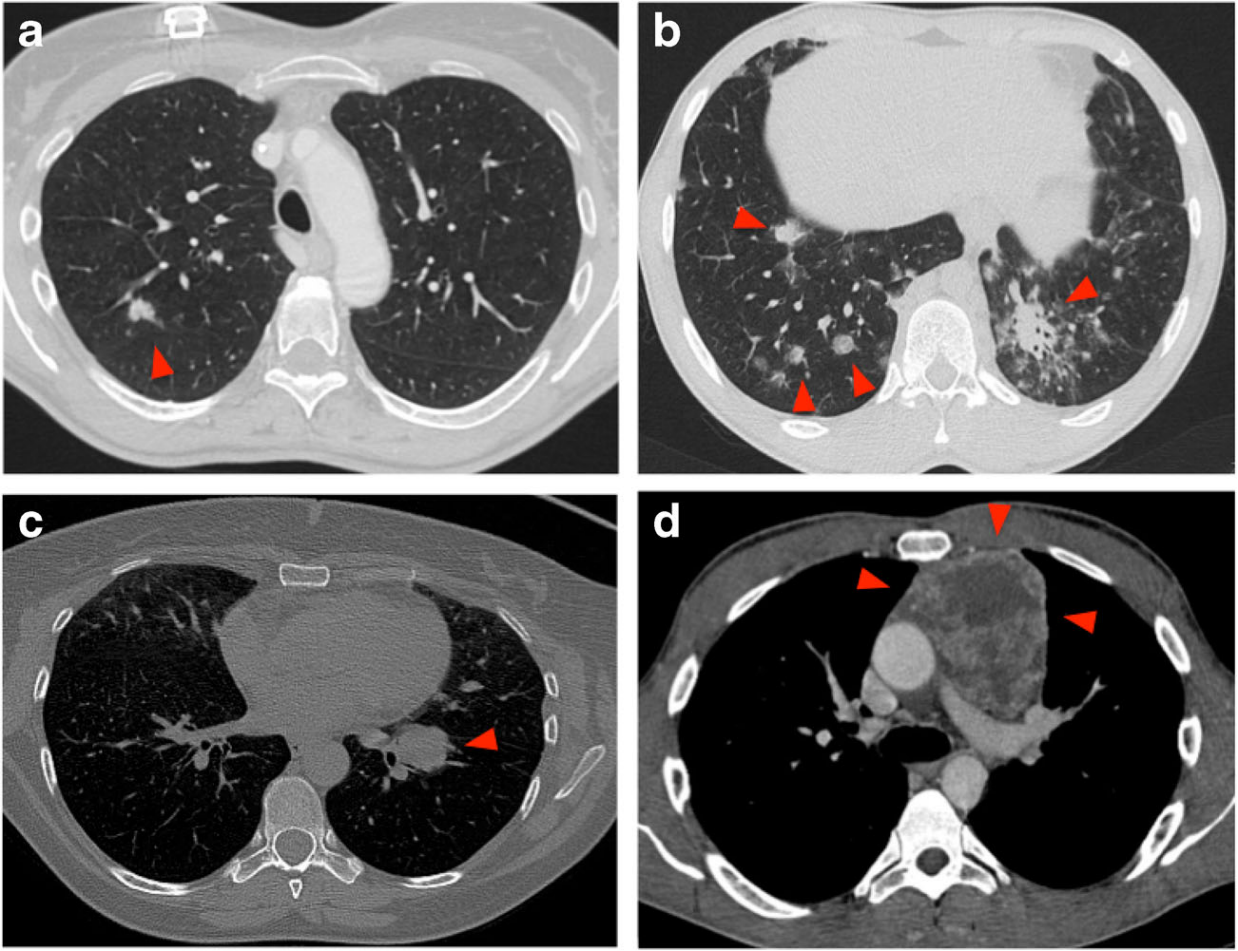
Indications for CT-guided chest biopsy. (a) Solitary pulmonary nodule. (b) Parenchymal infiltrates in which an infectious organism cannot be isolated. (c) Hilar mass following negative bronchoscopy. (d) Undiagnosed mediastinal mass
